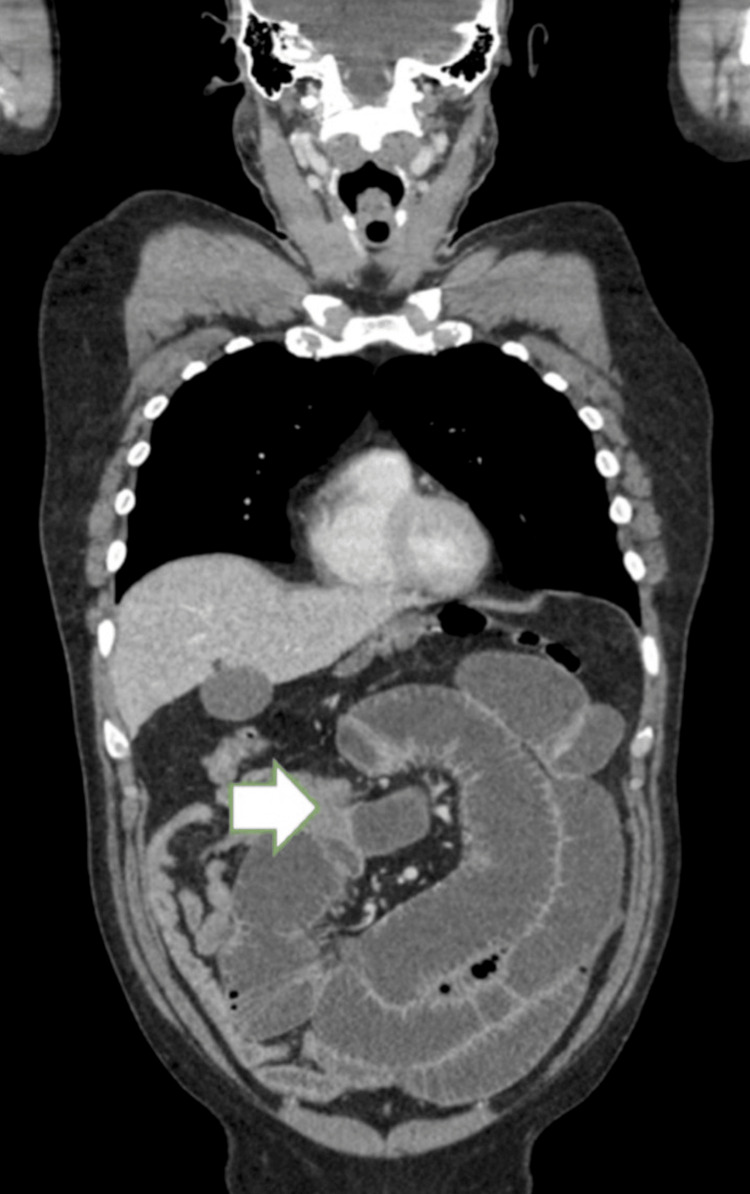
Contrast-enhanced CT abdomen and pelvis (coronal section) showing distal jejunal obstruction (white arrow)
